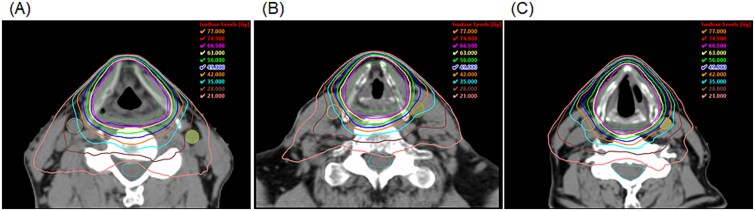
Dose distributions for the three representative cases. Axial CT images showing the PTV and carotid artery contours with isodose lines for (A) distant, (B) intermediate and (C) proximal cases.
